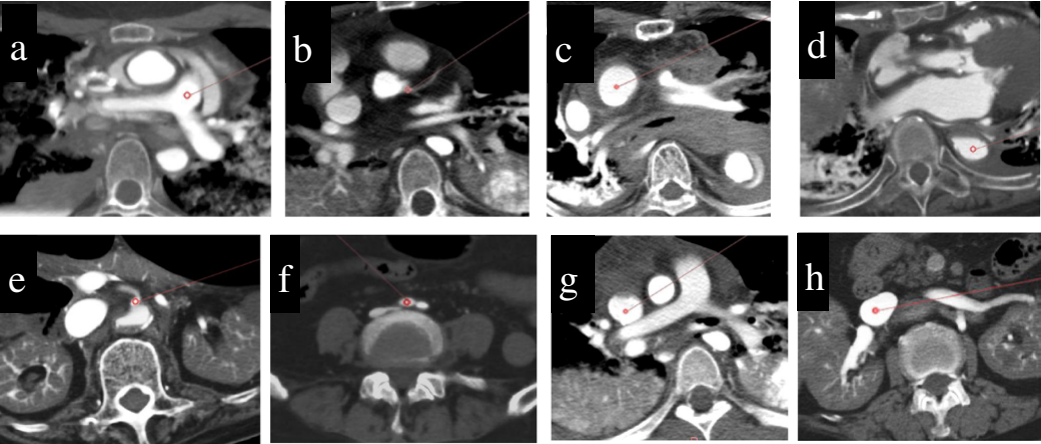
PMCT of 8 target blood vessels of a 64-year-old man. A red circle indicates a region of interest in which CT attenuation value (HU) was measured. a. Pulmonary artery trunk b. Left main coronary artery c. Ascending aorta at the level of the tracheal bifurcation d. Abdominal aorta at the level of the diaphragm e. Root of the celiac trunk f. Right common iliac artery immediately after branching from the descending aorta g. Superior vena cava at the level of bifurcation pulmonary artery. h. Inferior vena cava at the level of renal vein.
